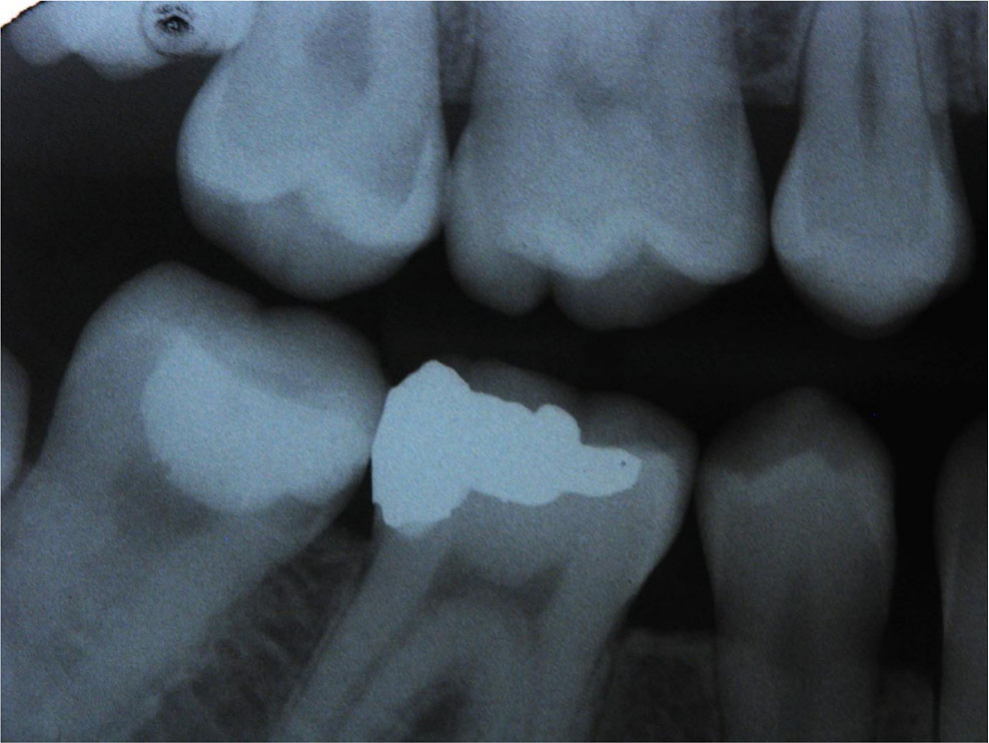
Radiograph showing caries mesially in the second upper molar
